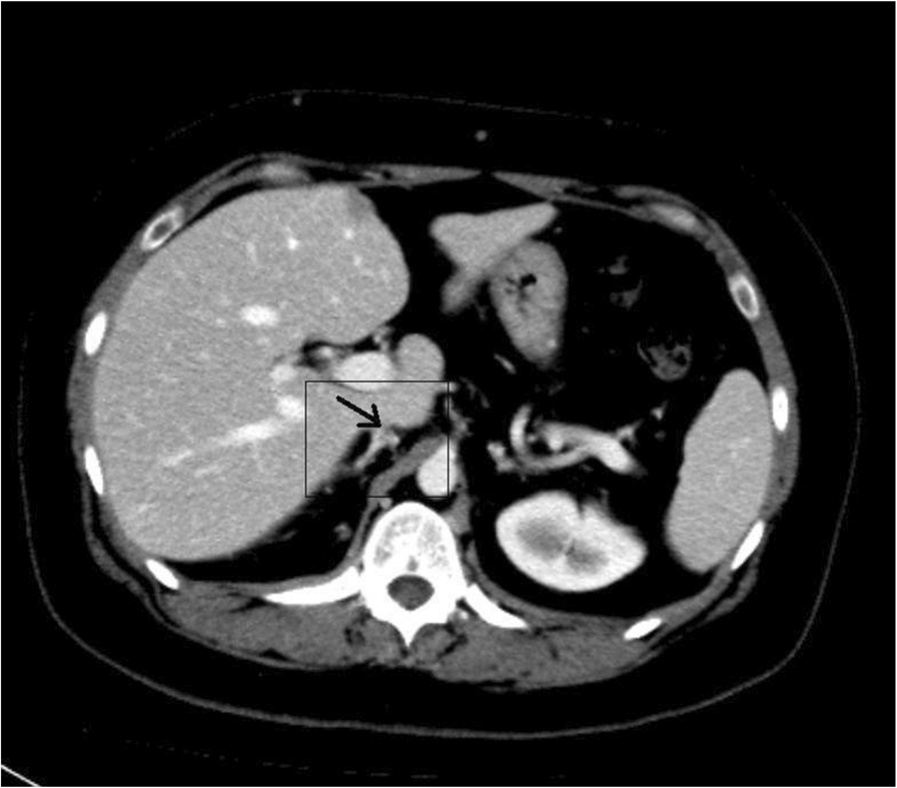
Computed tomography showed narrowed infra hepatic IVC (boxed region and arrow).
